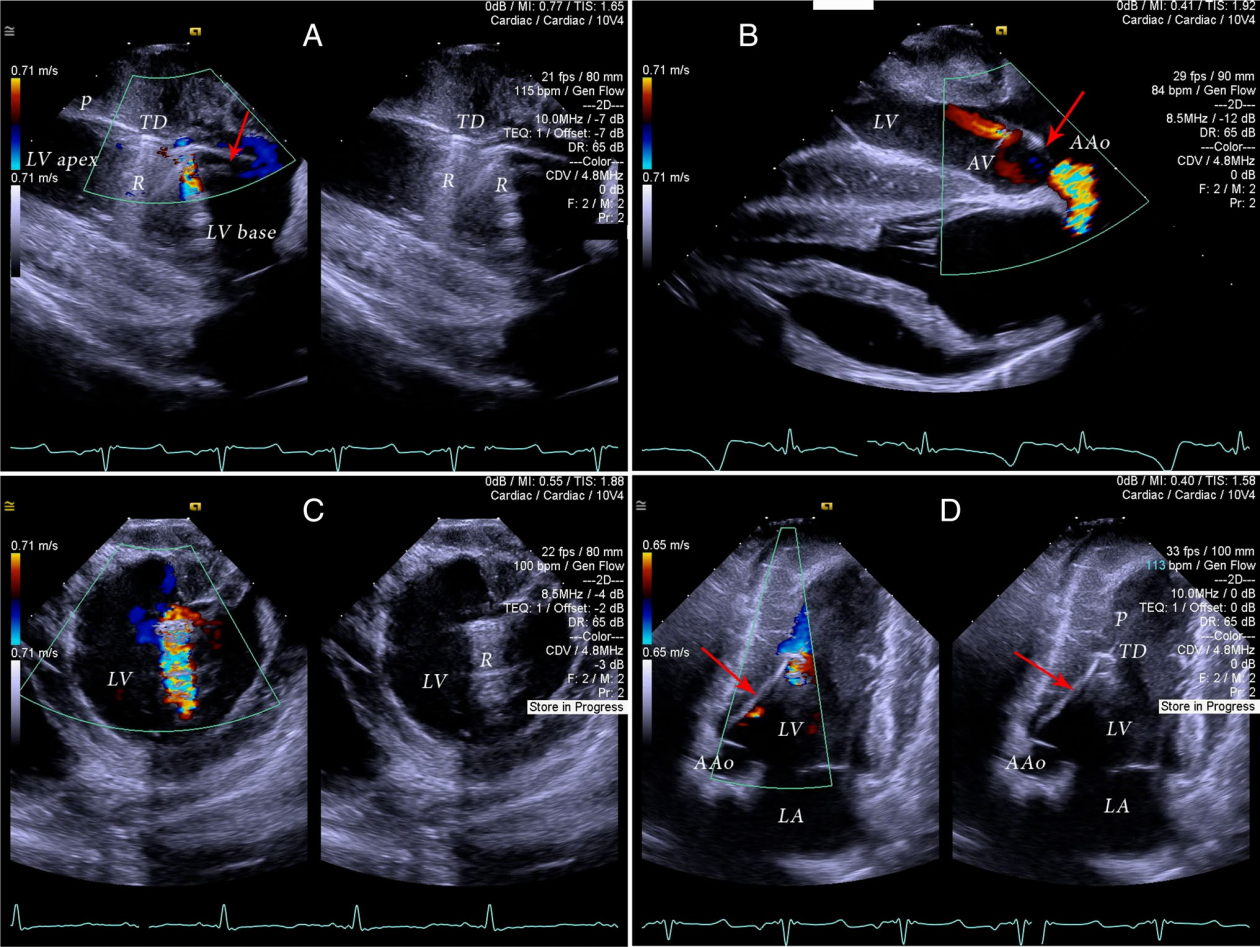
Two-dimensional grey-scale and color-Doppler imaging to confirm correct positioning of the Impella CP. LV – left ventricle, LA – left atrium, AAo – ascending aorta, TD – tea-drop part of the Impella, p- pigtail part of the Impella, R – reverberation artifact. Red arrow points to the Impella catheter traversing towards left ventricular outflow tract and aortic valve. A Longitudinal (in-plane) view of the correctly positioned Impella with Color Doppler inflow visualized within mid-LV. Extensive reverberation artifacts originate from the metal tea-drop and from the base of blood inlet area. B Longitudinal view of the correctly positioned Impella at the level of the aortic valve and aortic root. The view is an equivalent of inverted 120 TEE view. Color Doppler visualized blood outflow from Impella pump into the proximal ascending aorta. Mild aortic incompetence is visible due to the valvular leaflets coaptation restriction by Impella catheter. C Short axis left ventricular view at the level close to the LV base. Color Doppler demonstrated appropriate blood inflow site position of the Impella away from the mitral leaflets and subvalvular apparatus. D Apical view, an equivalent of transthoracic 3-chamber apical echocardiographic view. Color Doppler demonstrated adequate Impella blood inflow in mid-left ventricular cavity
